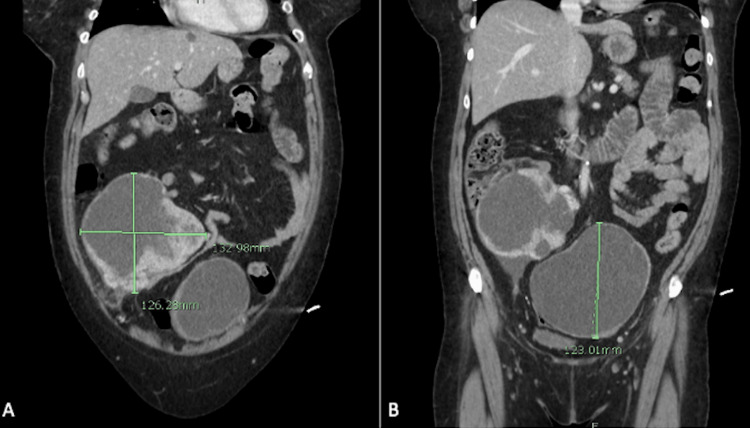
Coronal images of CT scan showing bilateral ovarian masses. A) Depicts the poorly differentiated thyroid carcinoma arising from a malignant struma ovarii. B) Depicts a serous cystadenoma with chronic inflammation and early fibrosis. CT, computed tomography
